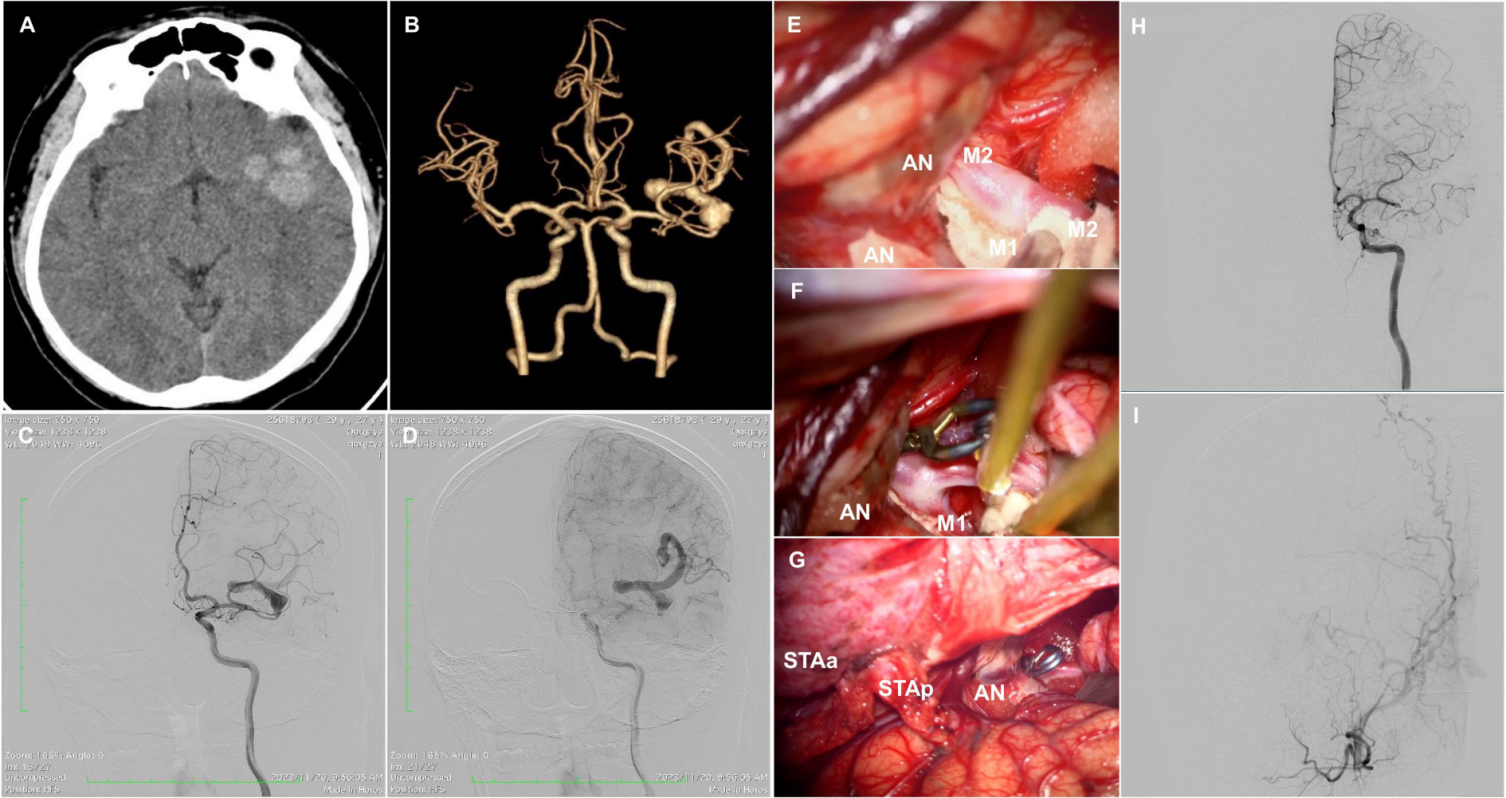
(A) Preoperative computed tomography (CT) showing a mixed-density, globoid mass in the left frontotemporal insula. (B) CT angiography (CTA) revealing a partially thrombosed giant serpentine aneurysm (GSA) of the left middle cerebral artery (MCA). (C) Early-phase digital subtraction angiography (DSA) demonstrating a serpentine vascular channel arising from the inferior trunk of the left MCA. (D) Late-phase DSA showing delayed filling of the serpentine channel supplying the distal MCA territory. (E) Intraoperative view of the proximal M2 inferior trunk identified proximal to the aneurysm. (F) Temporary clipping of the proximal M2 segment performed under neurophysiological monitoring. (G) Double-barrel superficial temporal artery–middle cerebral artery (STA–MCA) bypass followed by proximal parent artery occlusion. (H,I) Postoperative angiography seven days after surgery demonstrating disappearance of the serpentine lumen and excellent patency of both STA–MCA bypass grafts with distal filling of MCA branches.
